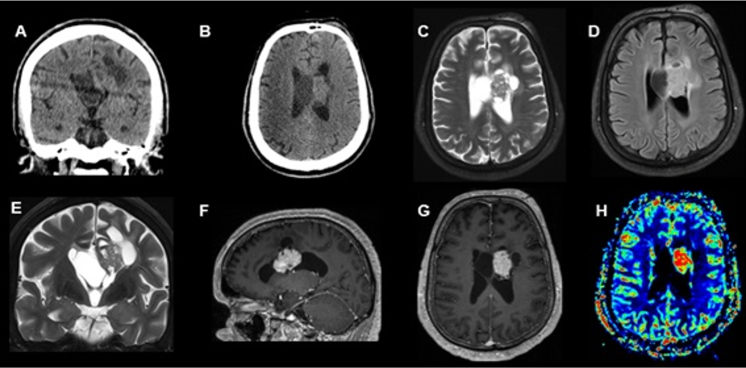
Coronal (A) and axial (B) nonenhanced brain CT shows a solid and cystic lesion within the lateral left ventricle and infiltrating adjacent white matter. Coronal and axial T2 (C, D), axial FLAIR (E) shows a well-demarcated, isointense and heterogeneous lesion with predominantly peripheral cysts located within the left lateral ventricle with infiltration of its lateral wall and the septum pellucidum. Sagittal and axial post-contrast T1 (F, G) sequence shows intense enhancement of the solid portion and increased rCBV on DSC perfusion (H).
